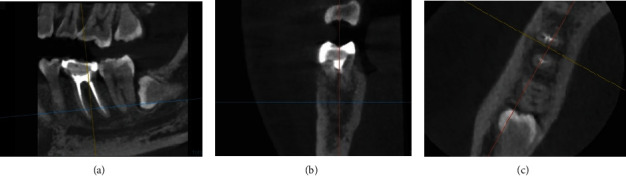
Cone beam computed tomography (CBCT) of Tooth #46 at 3-month follow-up. (a) Sagittal view, (b) coronal view, and (c) axial view.
